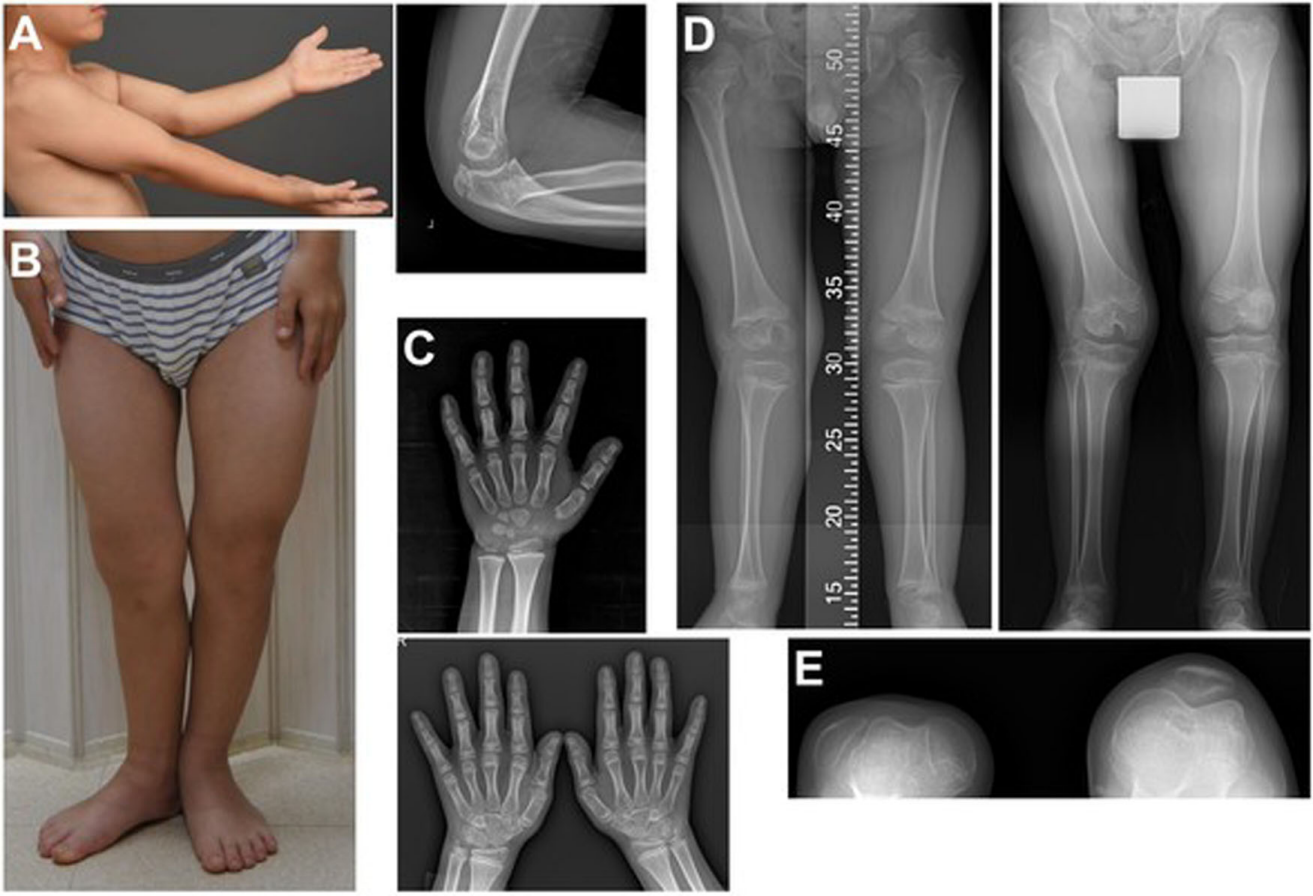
Photographs and radiographs of the extremities. A A photo taken at 11 years of age showing elbow flexion contracture and a radiograph showing radial head dislocation on the left side. B A photo taken at 11 years of age showing externally rotated lower extremities. The right genu valgum deformity was not visualized well due to an externally rotated position. C. A radiograph of the hand at 5 years of age (upper) barely showing any finding of the carpal coalition, and one at 11 years (lower) showing evident bilateral sites of carpal coalition. D, E Radiographs at 5 and 11 years of age showing aggravating genu valgum with the asymmetrical shape of the right distal femoral epiphysis and patellar dislocation.
